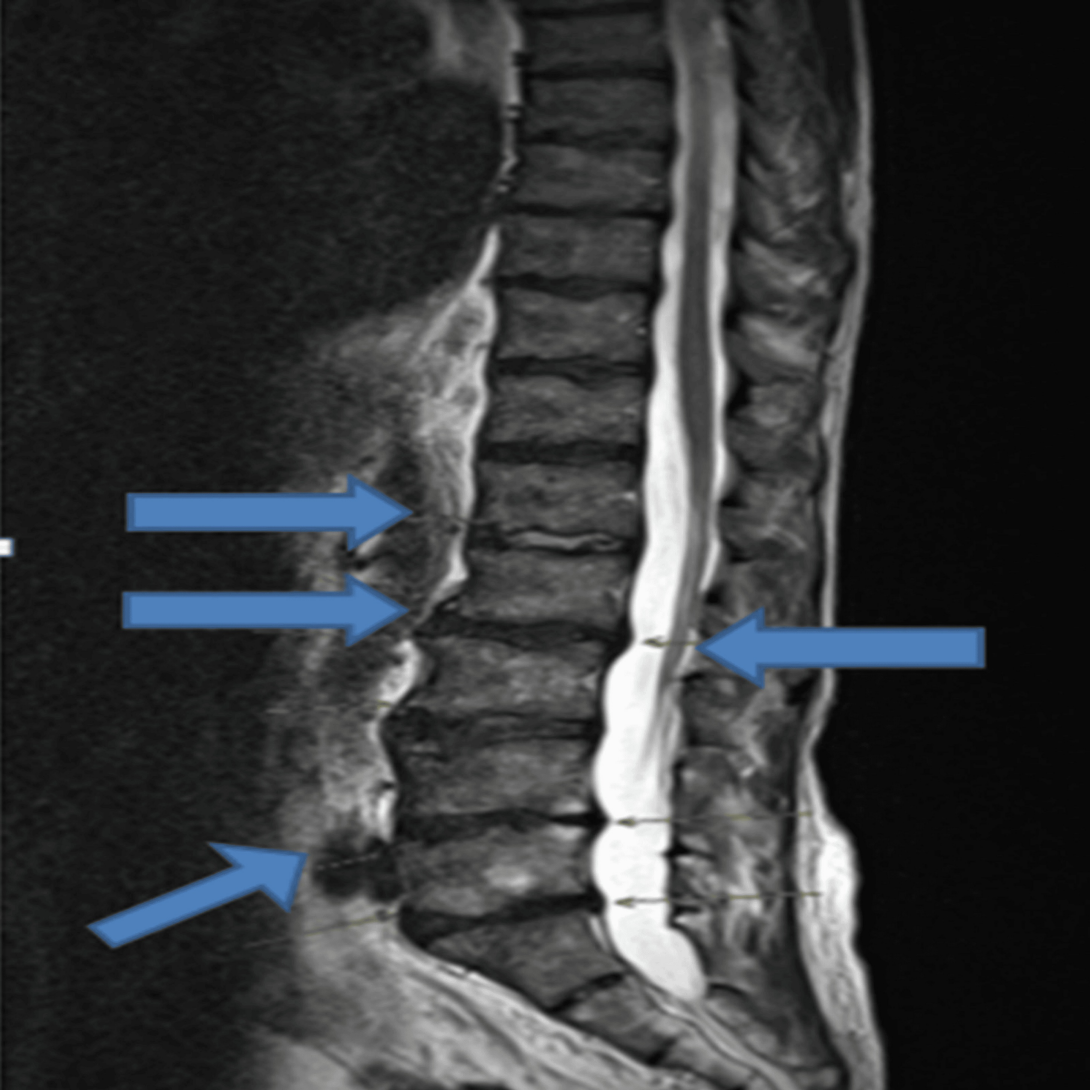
Whole spine MRI. Multiple blue arrows show stable multilevel spinal degeneration.
